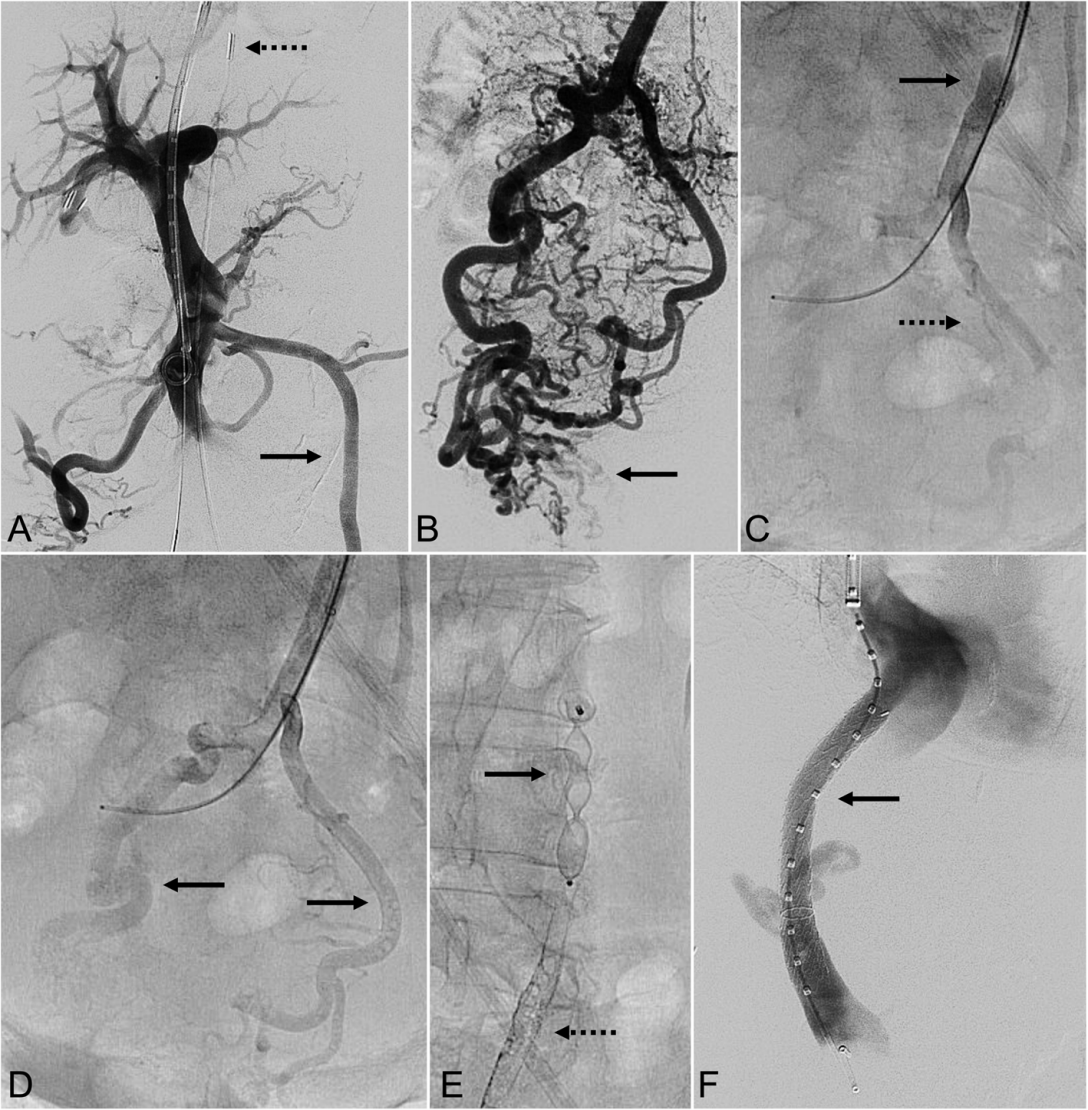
A *70-year-old female with bleeding rectal varices in the setting of non-alcoholic steatohepatitis cirrhosis. *A Digital subtraction portal venography following intravascular ultrasound-guided (dashed arrow) transjugular intrahepatic portal access, demonstrating retrograde flow down the enlarged inferior mesenteric vein (solid arrow). B Selective inferior mesenteric venography demonstrating extensive rectal varices (solid arrow). C Fluoroscopic image during sclerosant instillation with an occlusion balloon (solid arrow) inflated at the low inferior mesenteric vein trunk demonstrating sclerosant injection into the left superior rectal vein (dashed arrow). D Fluoroscopic image following further instillation demonstrating sclerosant in the bilateral superior rectal veins (solid arrows) and the rectal varices. E Fluoroscopic image demonstrating a 14 mm Amplatzer Vascular Plug II in the inferior mesenteric vein trunk (solid arrow) with the sclerosed vessels (dashed arrow). F Completion portal venography following transjugular intrahepatic portosystemic shunt placement with a 8–10 mm x 7/2 cm Viatorr TIPS stent dilated to 8 mm (solid arrow)
